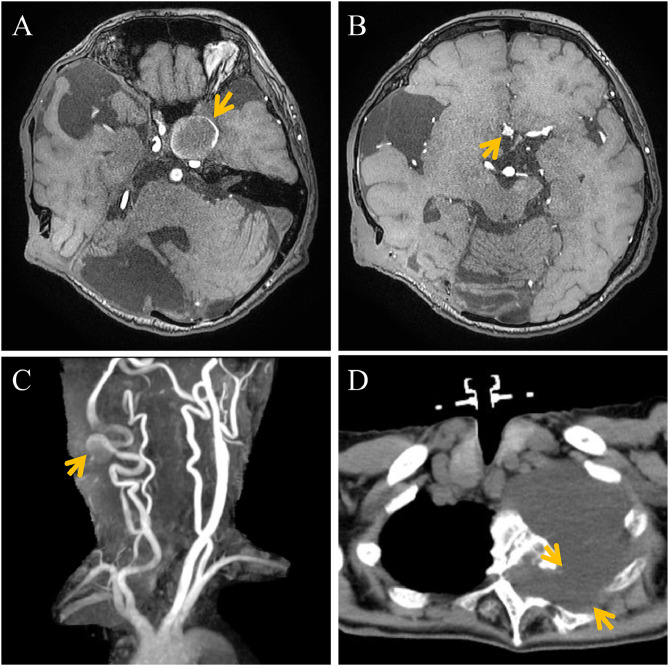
Preoperative images. (A-C) MR angiography, (D) Chest CT. (A) Left internal carotid artery (ICA) giant aneurysm, strongly compressing the left optic nerve and the cavernous sinus (arrow), a bony defect of the clivus and right posterior cranial fossa, an old infarction in the right cerebellum was observed. (B, C) Anterior communicating artery aneurysm (B, arrow) and cervical segment of the right ICA aneurysm (C, arrow) and marked arterial tortuosity were seen. (D) A meningocele protruding into the left thoracic cavity at the T3-4 level was identified (arrow). The left T3-4 intervertebral foramen was expanded secondary to a meningocele.
